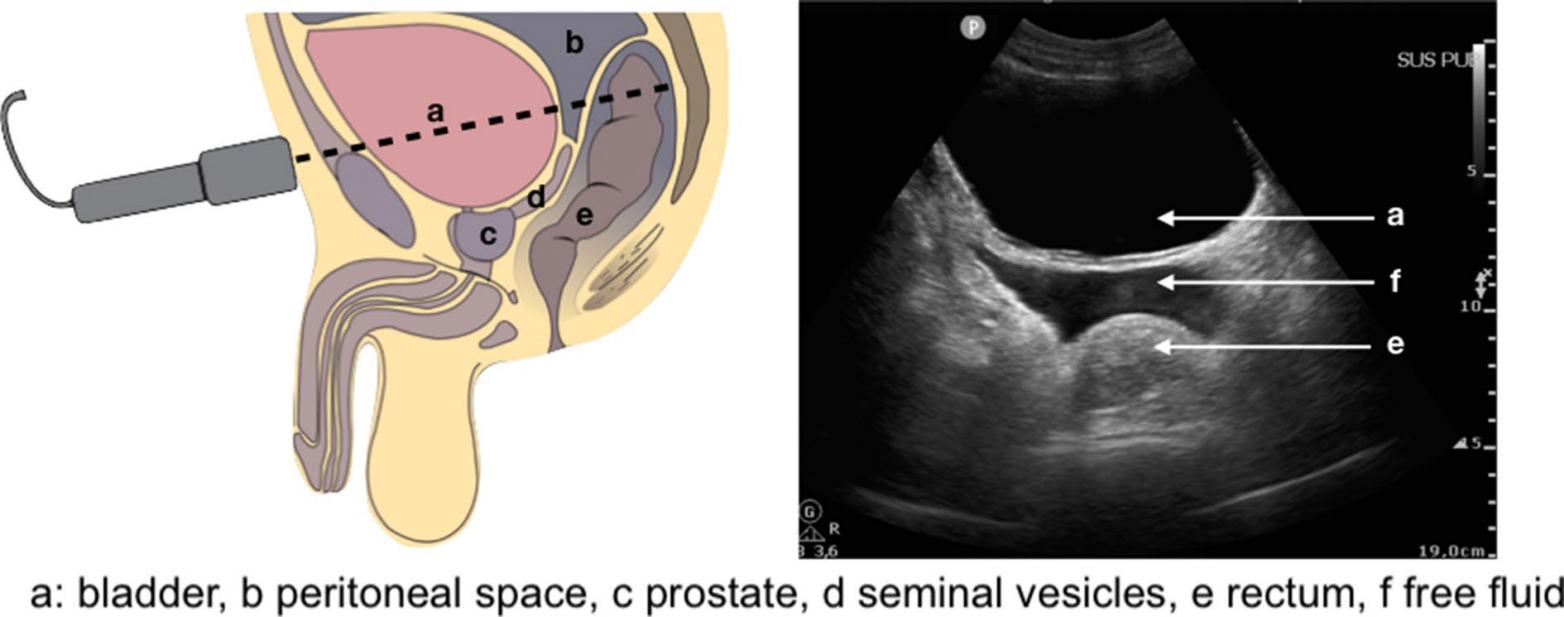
Transverse ultrasound view of the male pelvis with free fluid—probe oriented towards the peritoneal space
